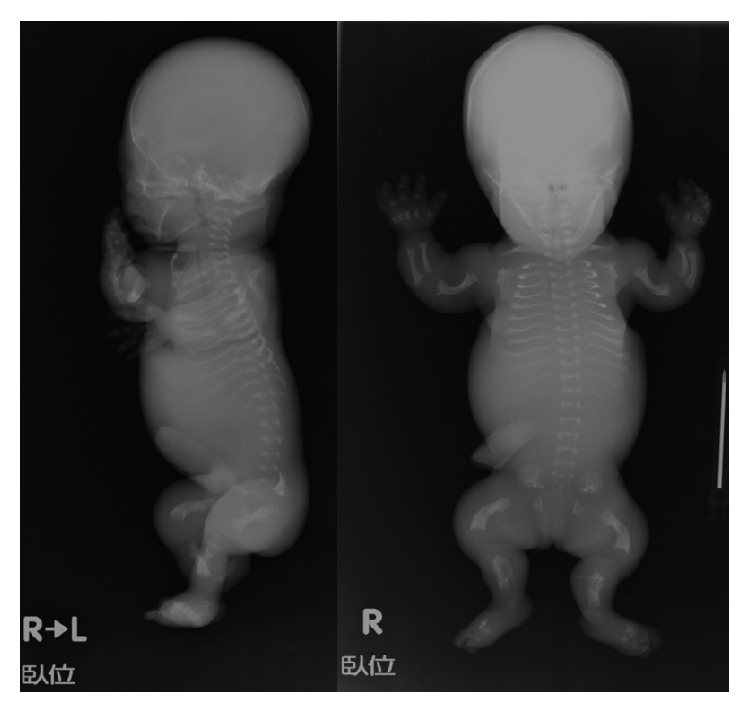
Anteroposterior and lateral radiographs of the fetus showing telephone receiver-like curved femurs and humeri with irregular metaphyses, H-shaped platyspondyly, and curved clavicles. No cloverleaf skull deformity was observed.
